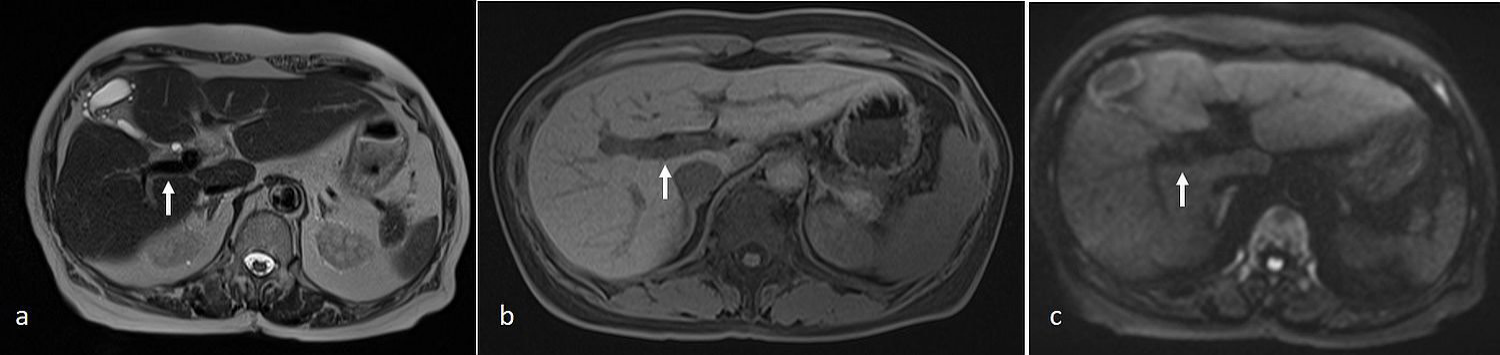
A 40-year-old gentleman underwent liver MRI for evaluation of raised serum alphafetoprotein on health screening. (a) Axial T2-SSFSE sequence showed normal flow-related signal void in the patent portal veins (arrow). (b) On T1 3D-VIBE, the portal veins appeared hypointense (arrow). (c) On high b-value DWI (*b* = 1000), the portal veins showed signal void (arrow). Portal veins were confirmed to be patent after intravenous administration of Dotarem (not shown). DWI, diffusion-weighted imaging; SSFSE, single-shot fast spin echo; VIBE, volume interpolated breath-hold examination.
